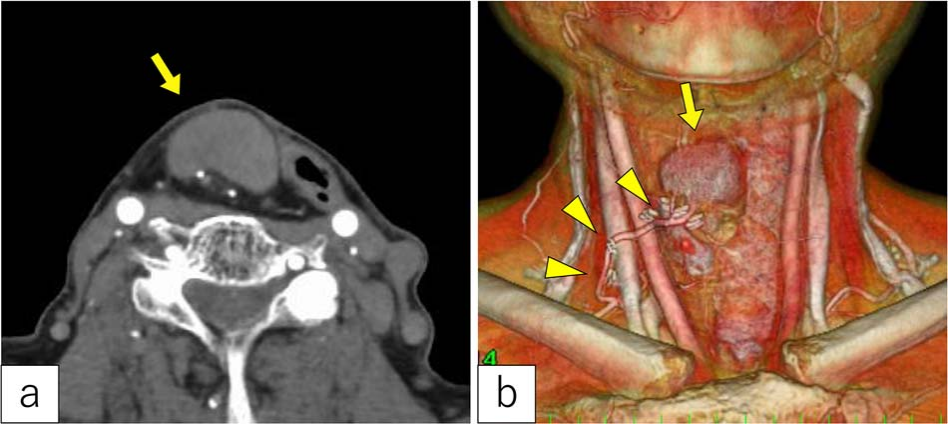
Radiological findings. (a) Computed tomography revealed a tumor under the cervical flap (arrow). (b) The tumor involved the vascular pedicle of the jejunal flap (arrowhead).
